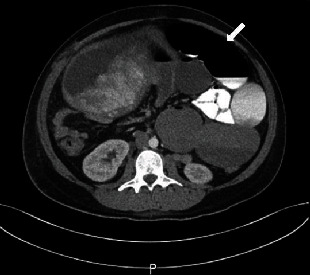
Small bowel obstruction demonstrated on CT. Severely dilated proximal small bowel (arrow) measuring up to 6 cm in diameter with abrupt tapering of the small bowel in the mid abdomen posterior to the dome of the uterus.
